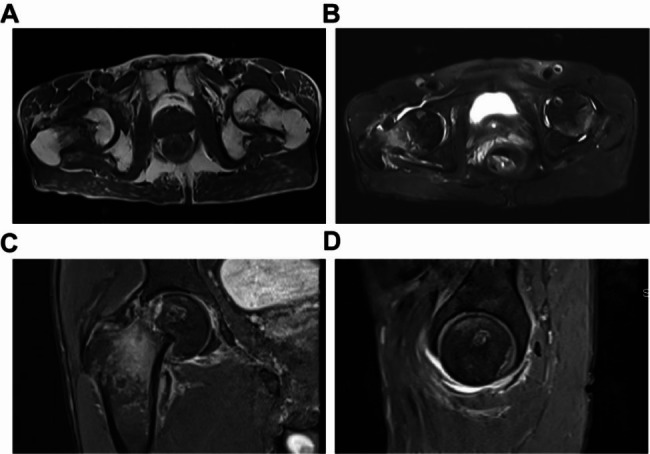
Hip joint MRI of a patient with first-time femoral neck fracture. (A) The T1-weighted image revealed low signal intensity in the right femoral neck and linear low signal intensity in the left femoral neck, which may be indicative of necrosis. (B) The T2-weighted image reveals the presence of subchondral edema in the right femoral head, high signal intensity in the femoral neck indicating femoral neck fracture, and local high signal intensity in the left femoral head without any evidence of trauma. (C-D) T2-weighted images revealed subchondral edema in both femoral heads, indicating osteonecrosis. There was also edema in the femoral neck on the right side, which was caused by a fracture of the femoral neck
